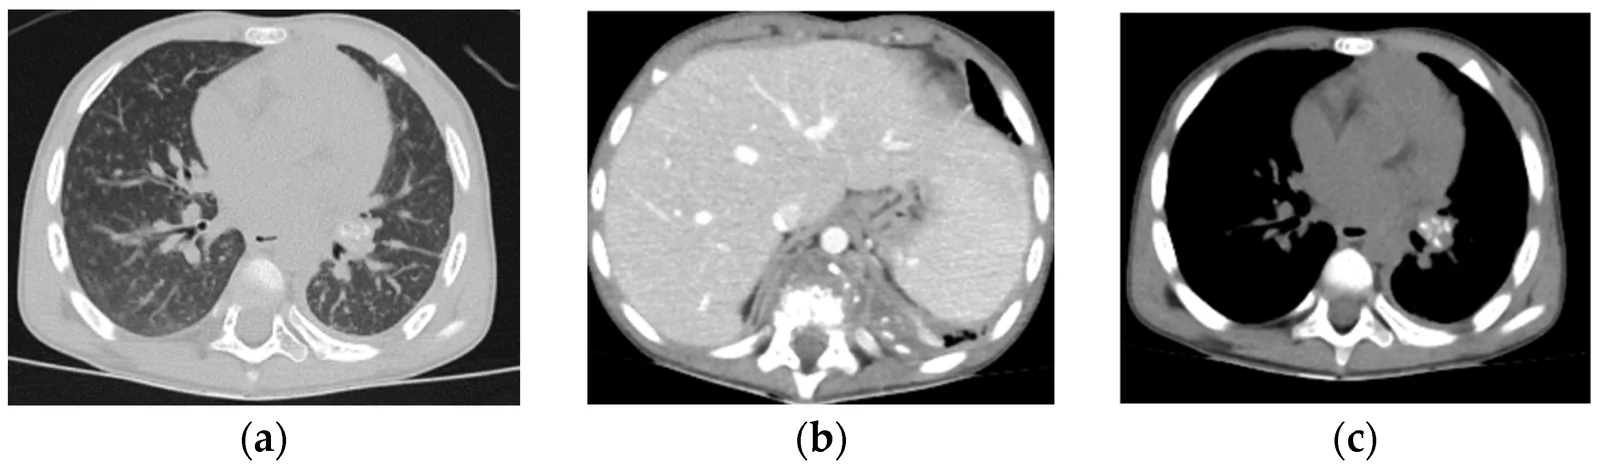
Contrast-enhanced chest and abdominal CT: (a) diffuse micronodular pulmonary lesions; (b) multiple splenic and hepatic micronodular lesions; (c) enlarged, partially calcified mediastinal lymph nodes.
